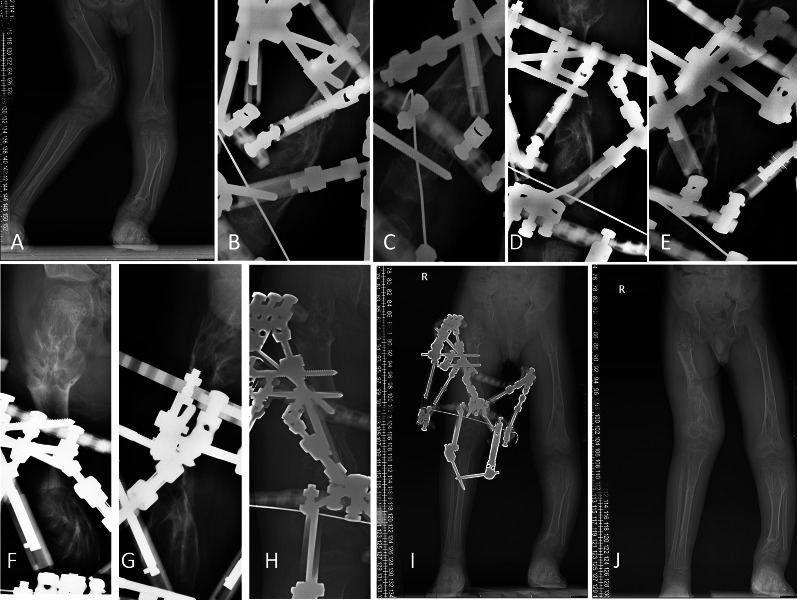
A 5Y1M boy (patient 9) with Ollier’s disease, right femur lengthened by 3 cm, 33°AC, with TSF fixator. A AP radiograph before operation. B, C AP and lateral radiographs during operation. D, E AP and lateral radiographs after 7 days of lengthening. F, G AP and lateral radiographs after 26 days of lengthening. H AP at the end of lengthening after 32 days. I AP before external fixation removal, 3.5 months after operation. J Full length AP 1 month after removal of the external fixator
